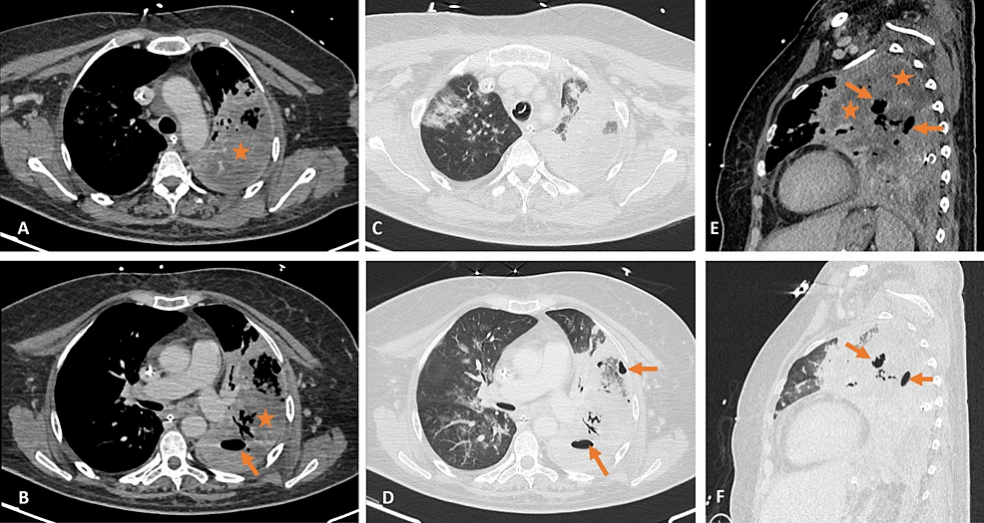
Chest computed tomography performed 11 days after hospitalization. Axial enhanced computed tomography images in a mediastinal window at the apex (A) and mid-lung (B) demonstrate heterogeneously enhancing left lung consolidation (asterisks), suggestive of pulmonary necrosis, with air foci (arrows) reflecting abscess formation. Axial enhanced computed tomography images in the lung window at the apex (C) and mid-lung (D) reveal increased left lung consolidation and air foci (arrows), in addition to right lung infiltrates. Sagittal reformatted images in mediastinal (E) and lung (F) windows depict the same findings.
